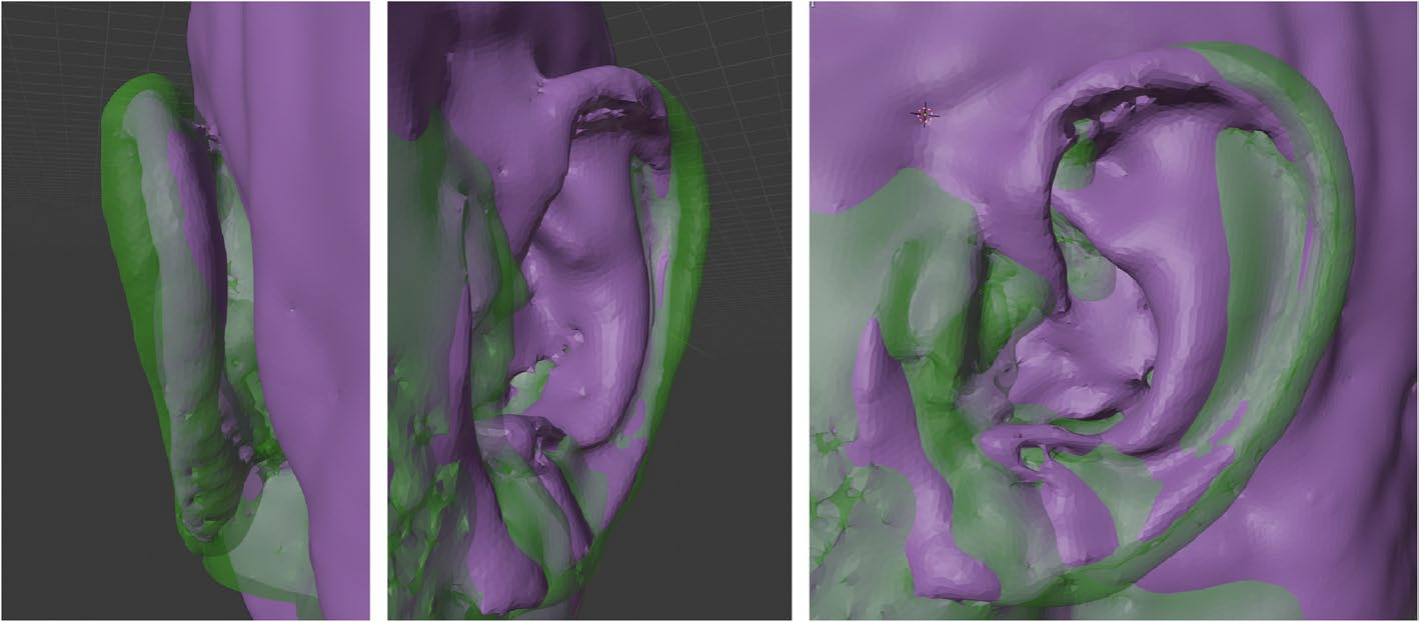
Image registration fusion of left and right outer ear from the MRI data (Fig. 3). Left side: posterior-anterior view; center: anterior-posterior view; right side: side-view. ‘Green’ represents data from the left ear, ‘purple’ indicates data from the right ear. Some asymmetries can be observed between the ears, which are rarely perceived in daily routine
